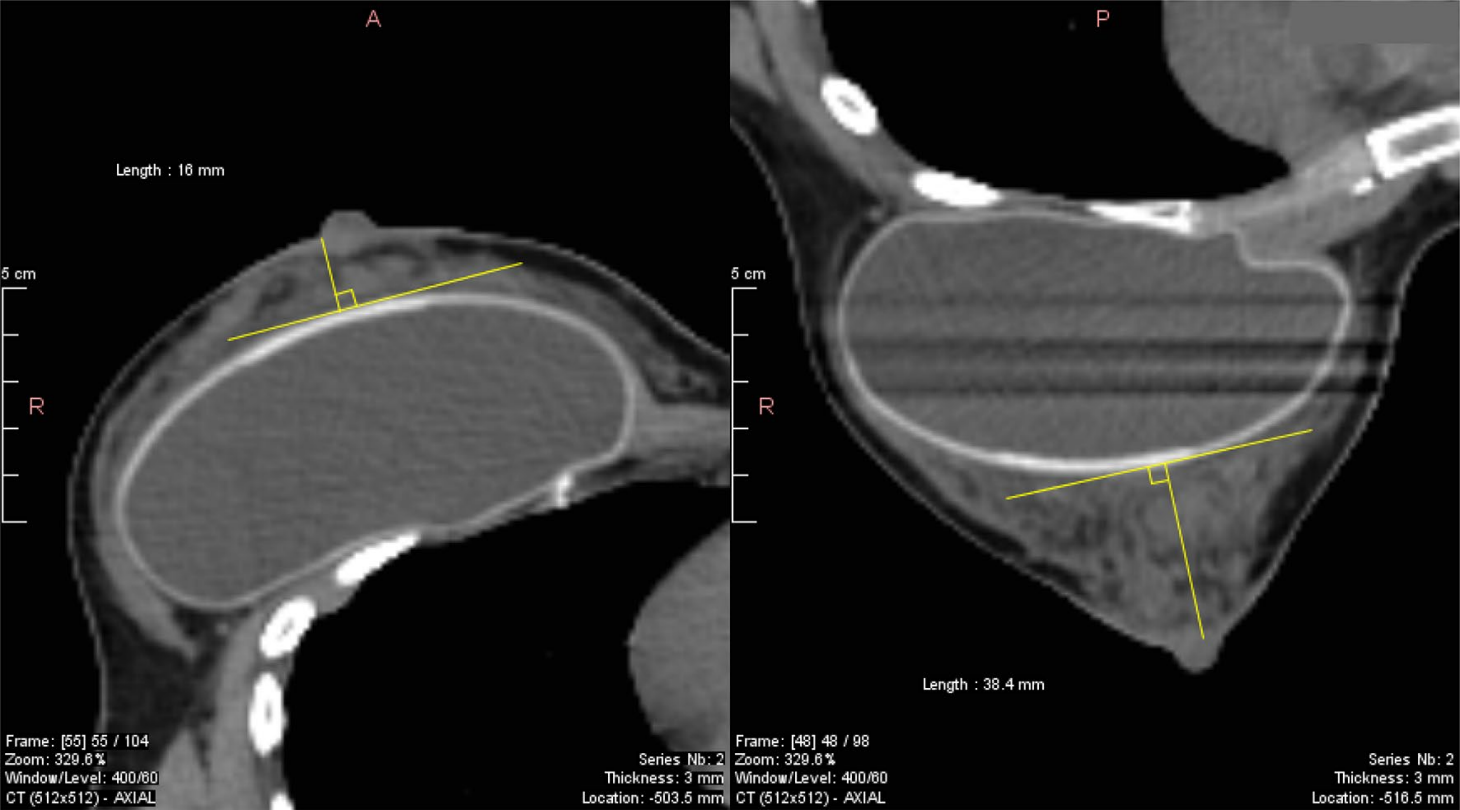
Breast augmentation implant supine and prone. Supine (left) and prone (right) CT of case 112, green color-coded in Figs. 3, 4 and 6. The breast depth measured from the pleura was 71 mm supine vs. 94 mm prone. The depth remeasured from the implant anterior surface is 16 mm supine vs. 38 mm prone, indicating a shift with gravity of > 50%.
